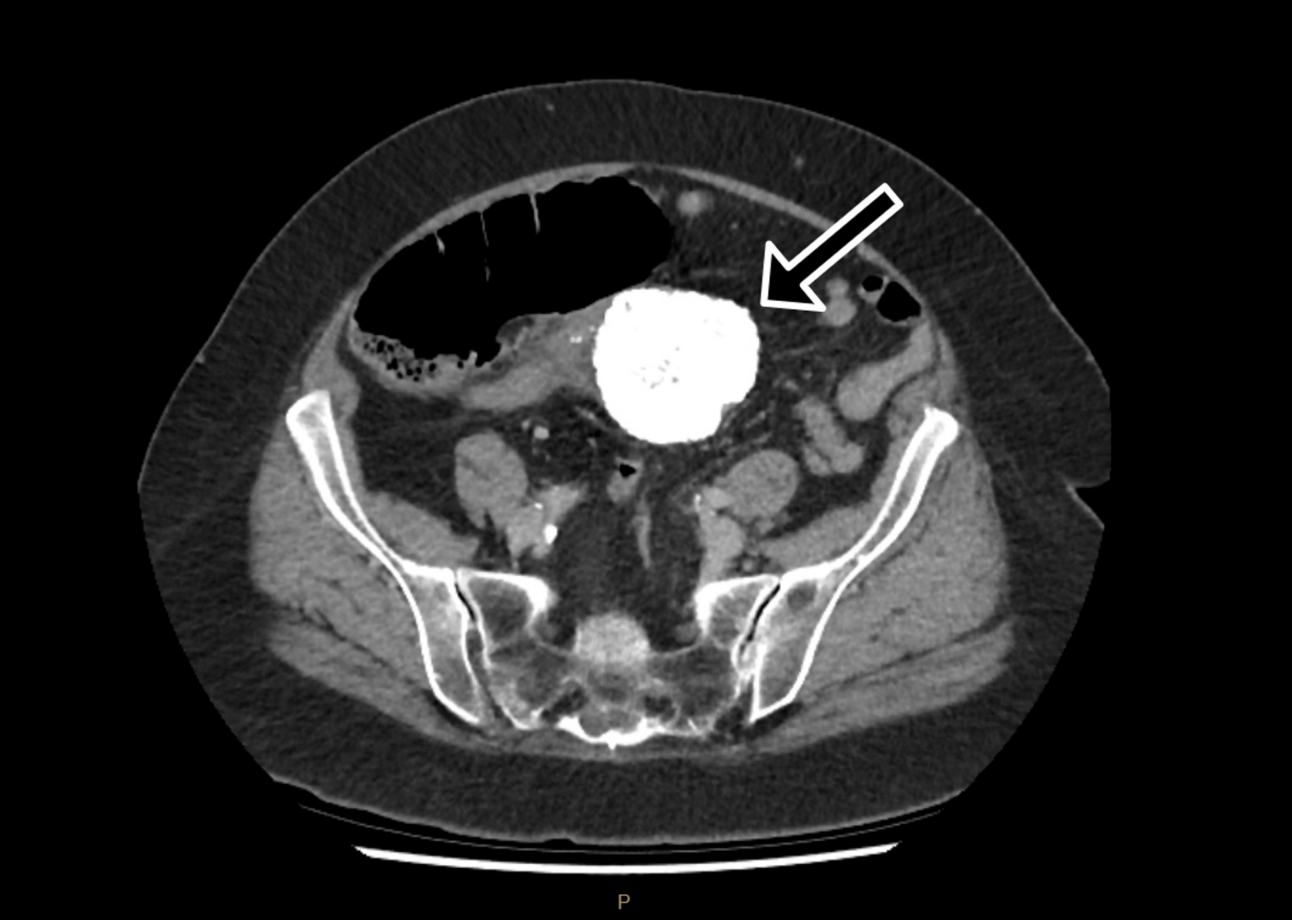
Axial portal venous phase CT of the abdomen demonstrating a large calcified central abdominal mass (arrow) causing proximal colonic dilatation consistent with large bowel obstruction CT: computed tomography
